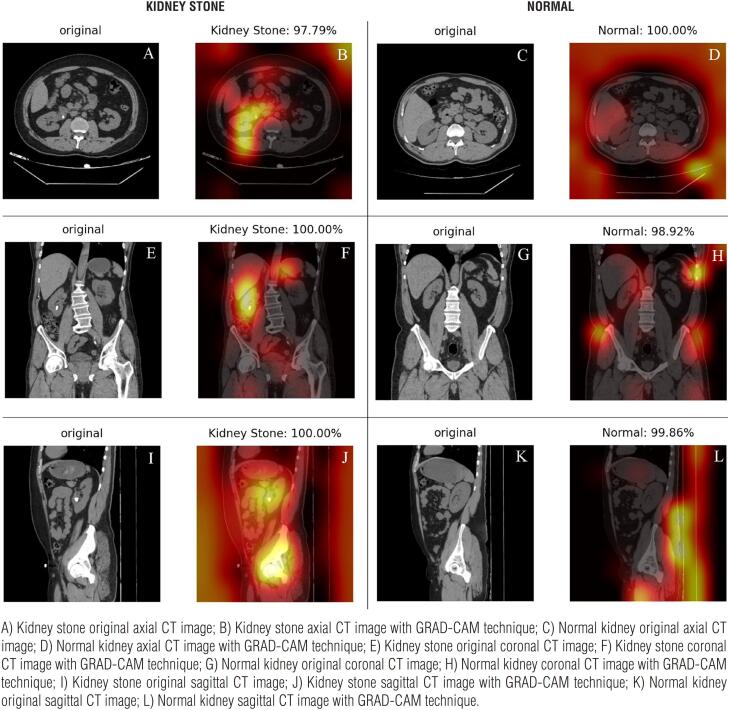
Axial, sagittal, and coronal CT images of patients bearing 0–1-cm-sized kidney stones and without kidney stones, demonstrated with the Grad-CAM technique. Percentages refer to the estimates of the AI model.
